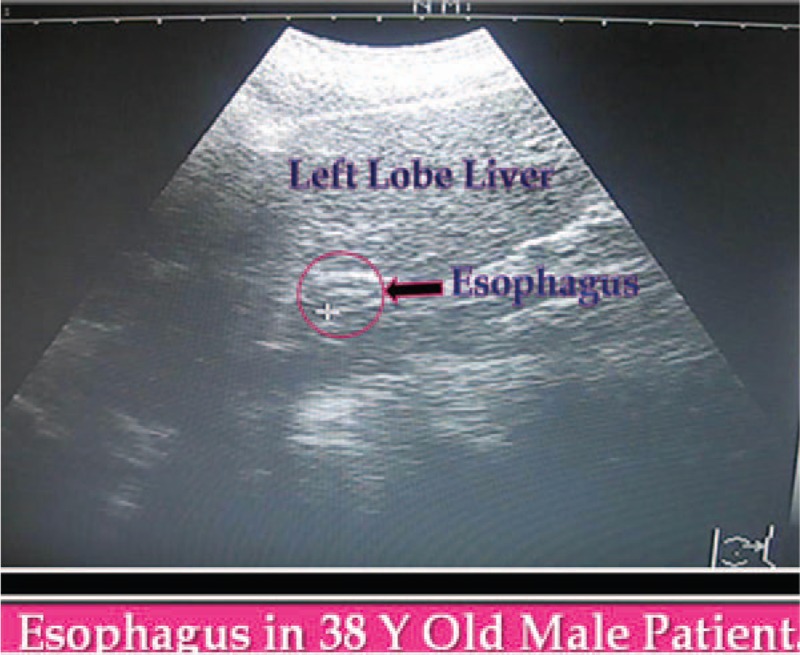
Conventional 2D US showing intra-abdominal portion of esophagus in male patient 38 years old. US = ultrasound.
